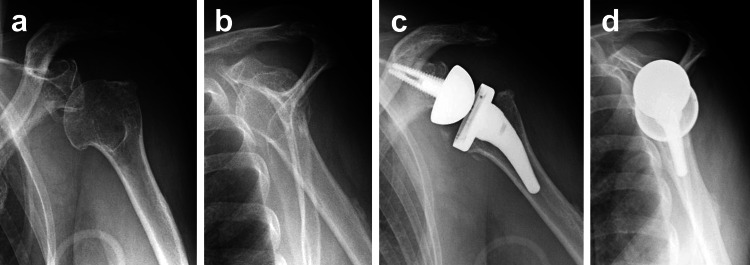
Plain radiographs The patient underwent reverse shoulder arthroplasty (RSA) for residual fracture-dislocation of the shoulder. Preoperative (a: anteroposterior image, b: lateral image) and postoperative (c: anteroposterior image, d: lateral image) radiographs.
